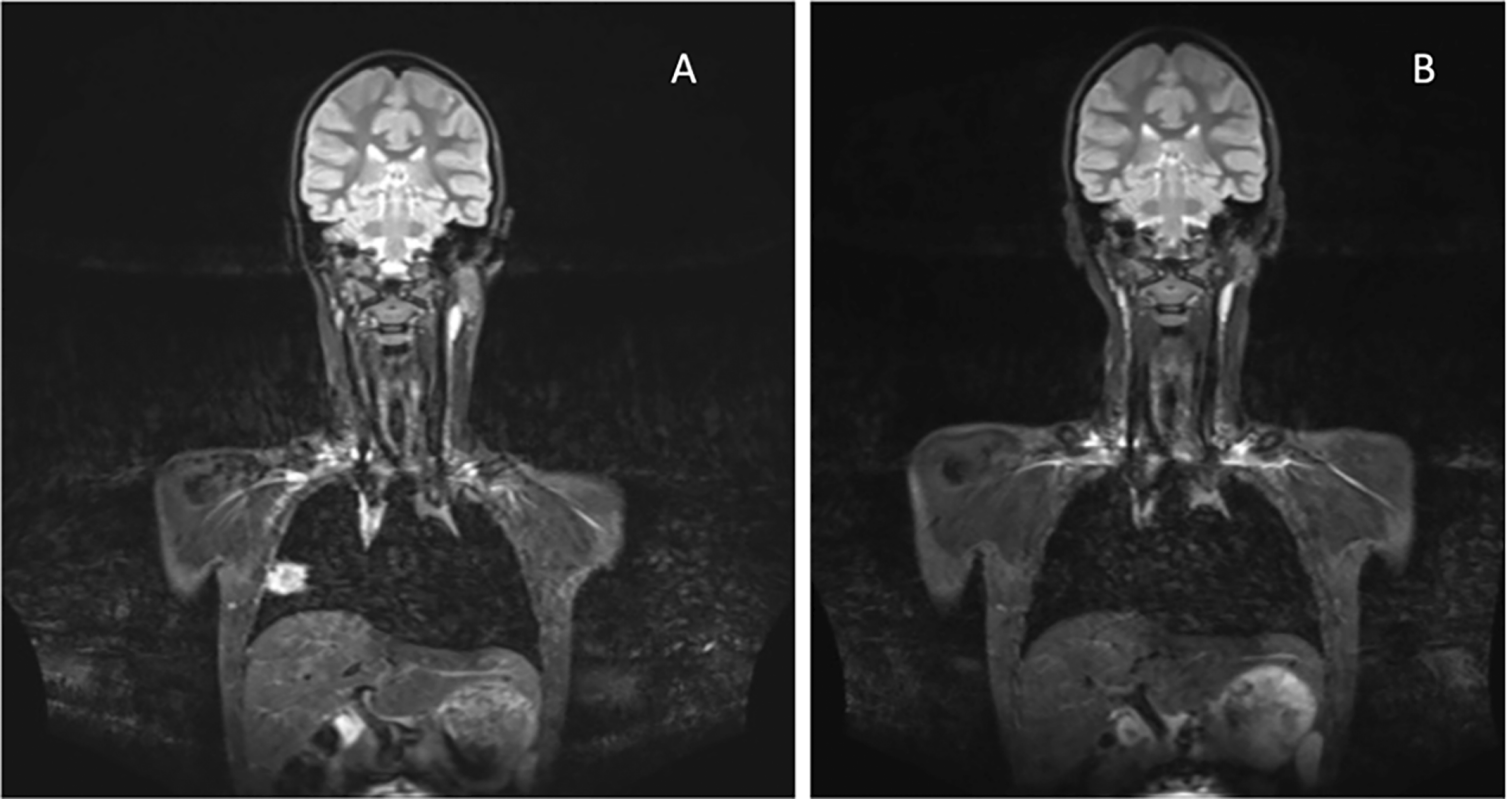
Pulmonary CNO lesions in an 8‐year‐old girl. (A) Coronal STIR MRI sequences reveal a pulmonary lesion in the right middle lobe measuring 2.8 × 2.3 cm, abutting the pleura. (B) Follow‐up MRI in the same patient, 11 months later, shows complete resolution of the pulmonary lesion under treatment with ibuprofen. CNO, chronic nonbacterial osteomyelitis; MRI, magnetic resonance imaging.
